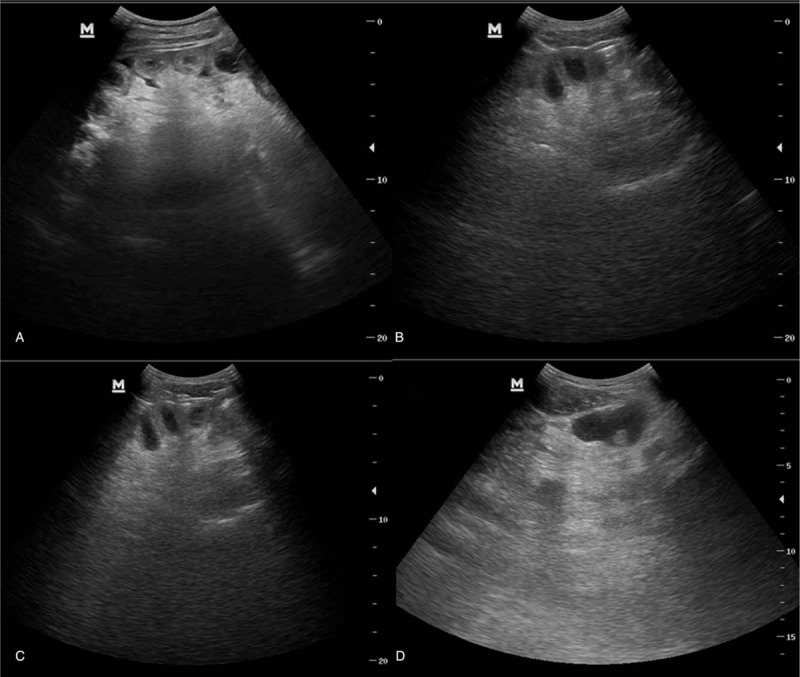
Bedside abdominal ultrasonography scan. (A) indicates intestinal cavity expansion and severe intestinal wall edema in this patient on July 21, 2017. (B) and (C) show that the intestinal wall still had significant edema on July 23, but the tension was reduced. (D) shows that the intestinal wall edema and tension were significantly reduced by July 25.
